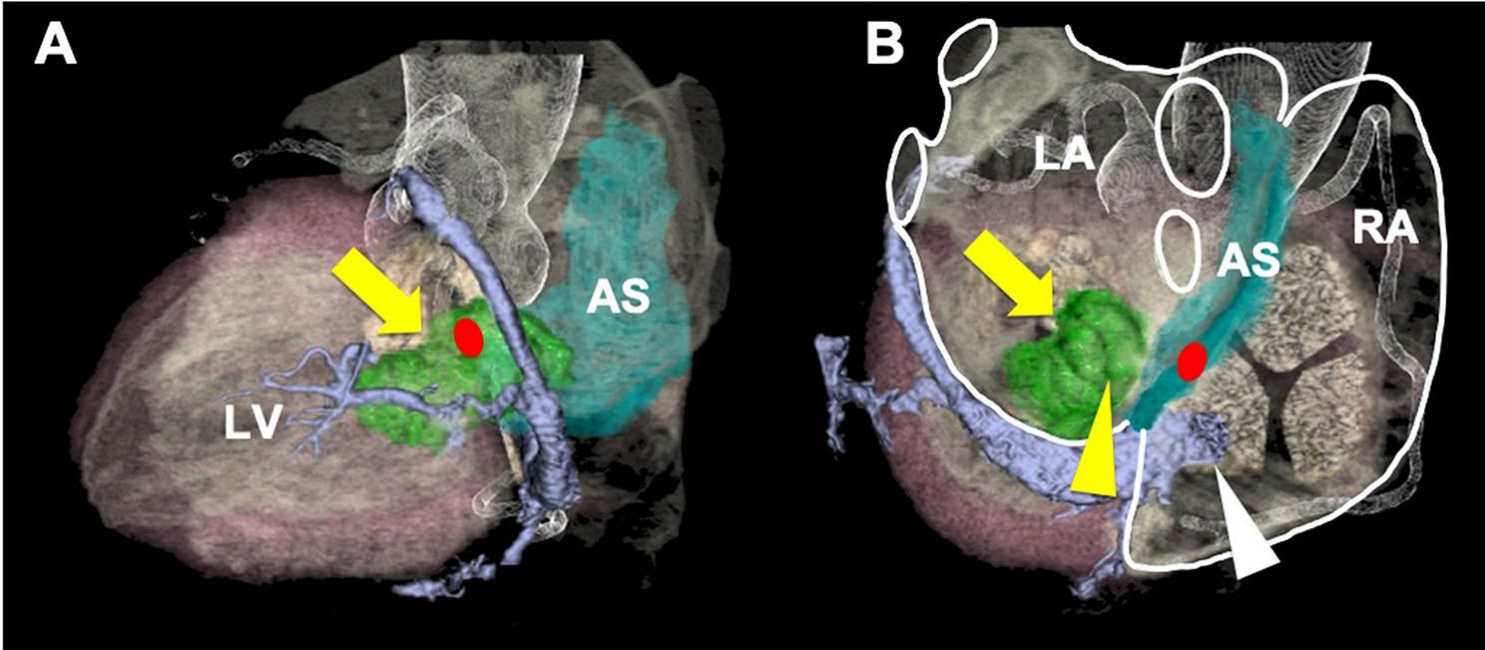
Cardiac CT. The left atrial tumor (yellow arrow) had no significant nutrient vessels. Its stalk was attached to the posterior wall of LA (yellow arrowhead). This tumor is close to the coronary sinus and its ostium (white arrowhead) and atrioventricular node (red circle). CT: computed tomography, LV: left ventricle, AS: atrial septum, LA: left atrium, RA: right atrium
